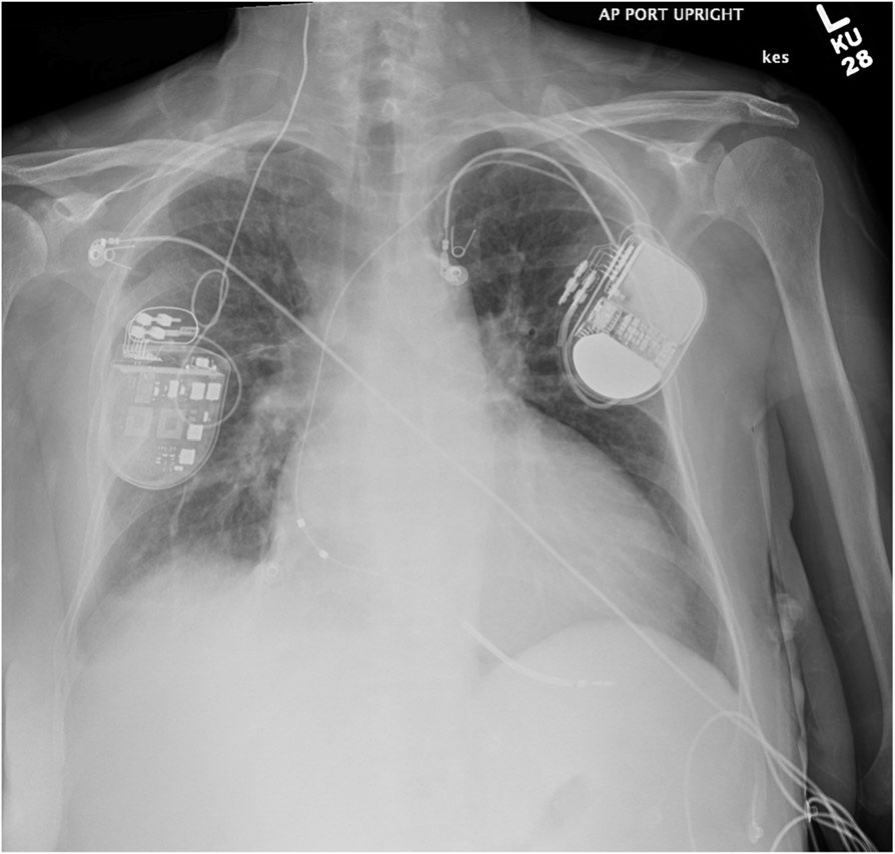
Chest X-ray. Post-implantation of Barostim NEO device with intact right carotid anchored suture wing of the non-isodiametric lead
